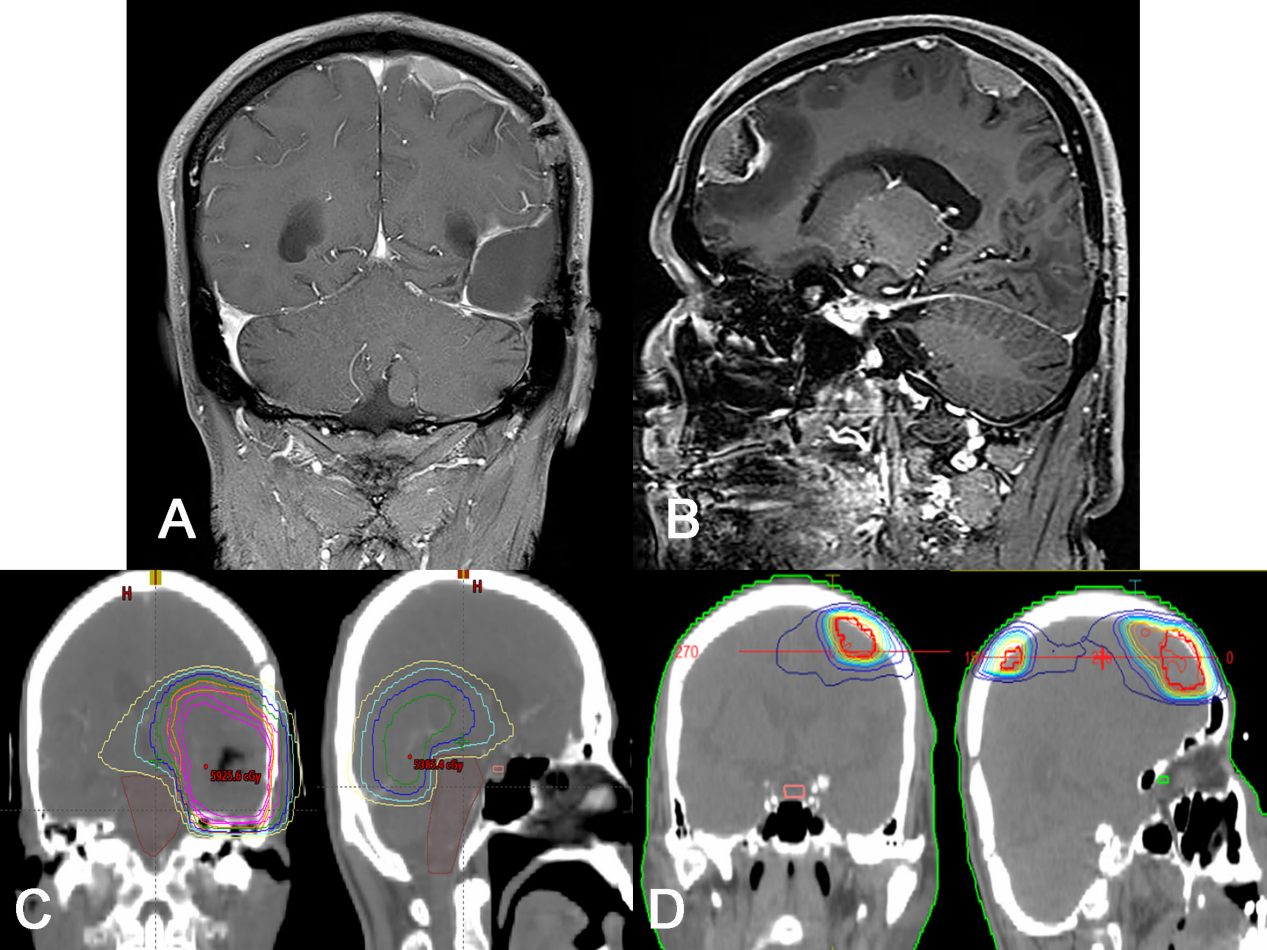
Radiological and treatment plan images for recurred lesions. (A, B) Gd-enhanced T1-weighted MRI showing two enhanced, dural based masses along the dura mater of remote site. Note that there is no definitive recurrence on the initial lesion. (C, D) Representative photos of dose plans for initial radiation (C, 60 Gy) and re-irradiation (D, 50 Gy) treatments. Note that there is no definitive overlap in radiation field in two dose plans.
